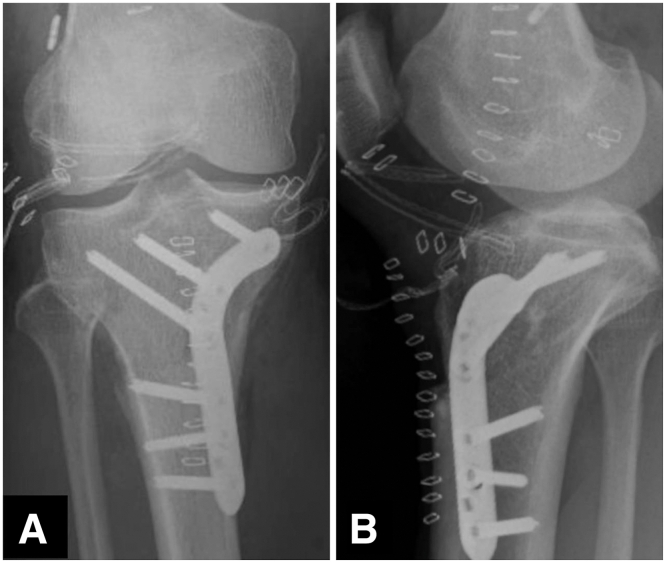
Immediate postoperative radiographs (standard knee x-ray). (A) Anteroposterior view. (B) Lateral view.
